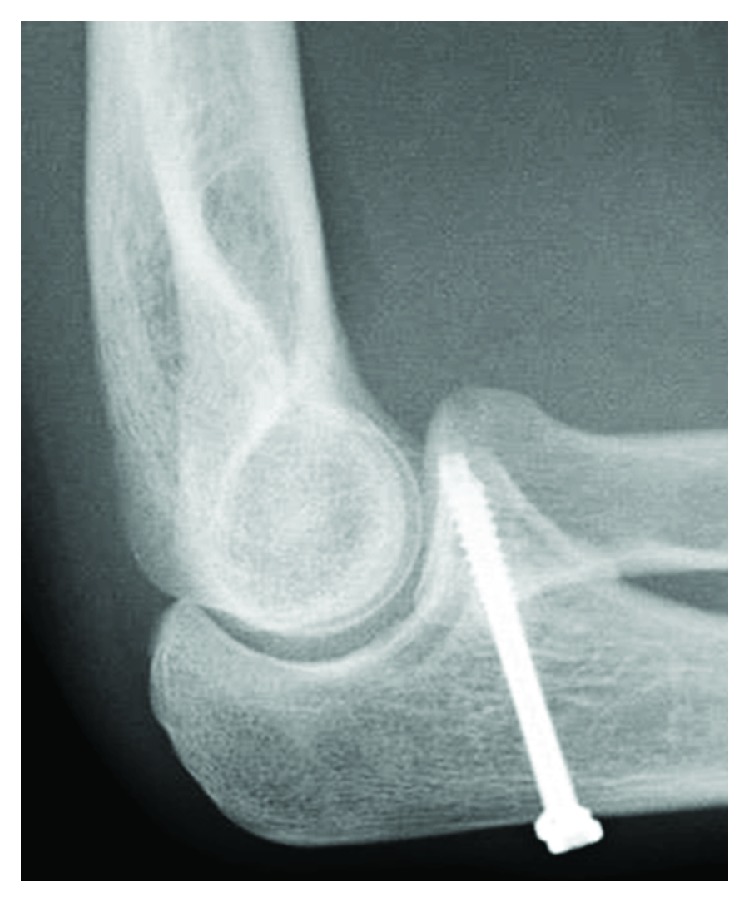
Case 2: postoperative radiograph shows fixation by two CCSs.
